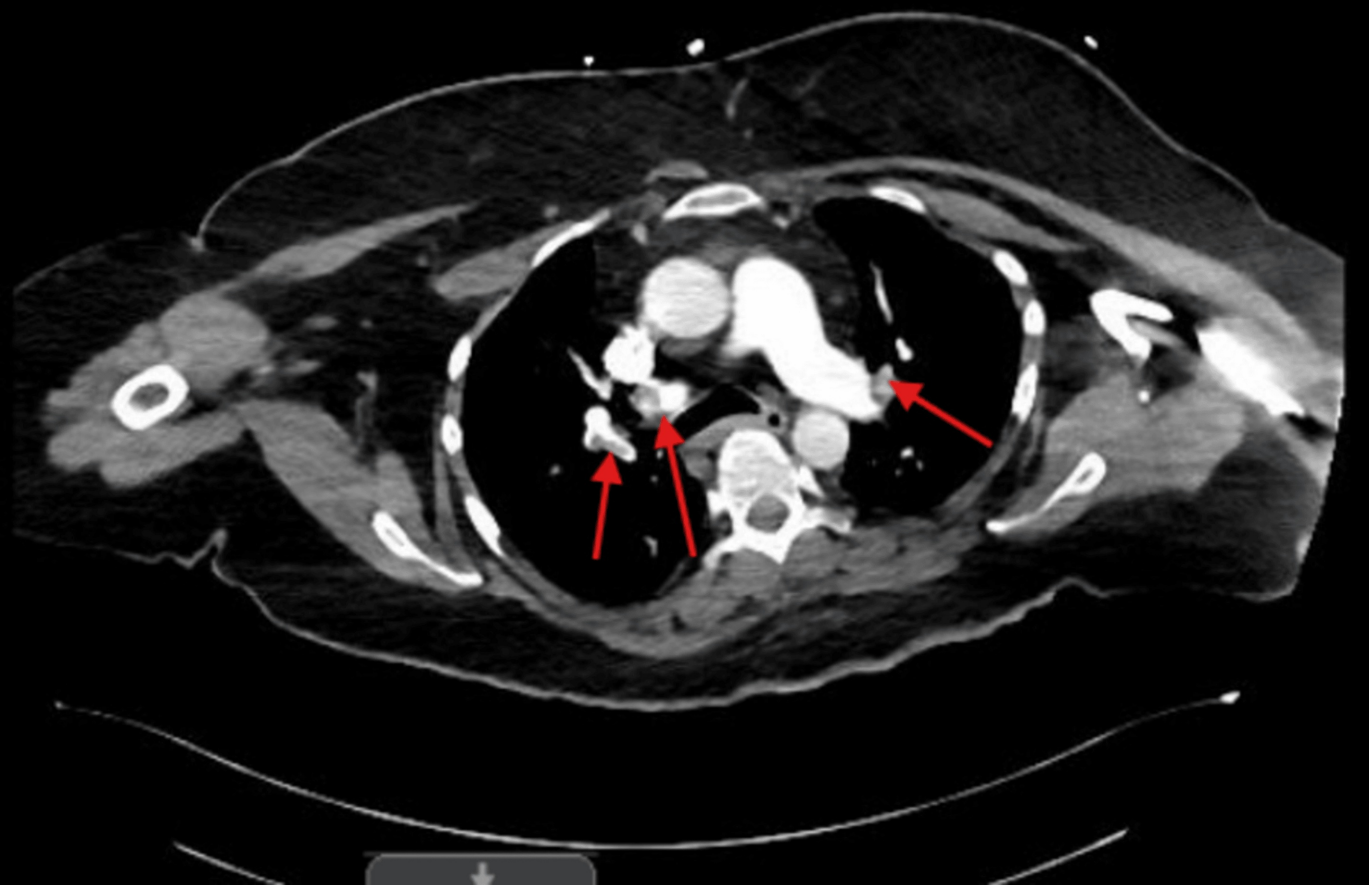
CT chest angiogram revealing bilateral segmental and subsegmental pulmonary emboli in the upper-mid lobes of the lung, which are depicted by the red arrows.
